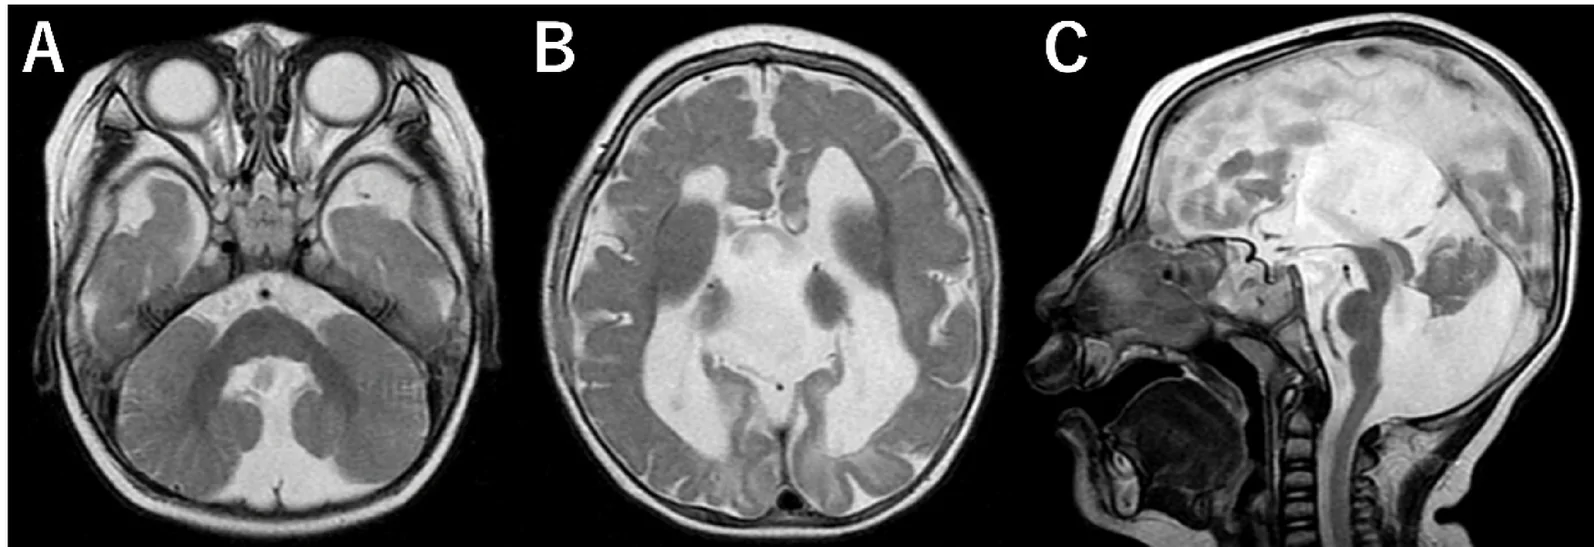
A, B: T2-weighted axial images, and C: T2-weighted sagittal images taken at age 8 months, showing cerebellar vermis hypoplasia (A, arrow), basal ganglia malformation (B, arrow), and corpus callosum dysplasia (C, arrow). The cerebellar tent is deviated upward and the posterior fossa is enlarged (C, arrowhead)
